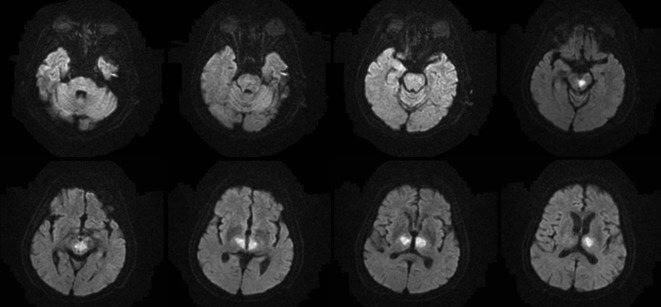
Diffusion-weighted MRI performed on the next day demonstrated hyperintensities in the bilateral thalami and rostral mesencephalon, consistent with restricted diffusion secondary to an acute ischemic stroke in the AOP territory.
